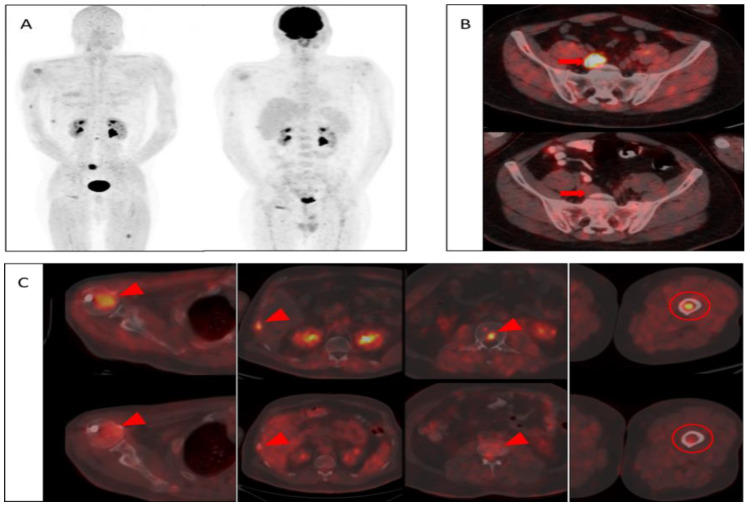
Patient No 3. 39-year-old male diagnosed with MM. (A) Visual comparison of maximum intensity projections of both modalities showed superior image quality and higher specific activity retentions in [68Ga]FAPI PET/CT (Left) without non-specific diffuse medullary uptake as in [18F]FDG PET/CT (Right). (B) In axial plane evaluations, both imaging methods demonstrated extramedullary involvement of MM in the presacral lymph node (Arrows); however, SUV_max_ value of the lymph node were noted to be significantly higher in [68Ga]FAPI PET/CT (Upper row). (C) In the visual evaluation of the bone lesions (Arrow heads), [68Ga]FAPI PET/CT (Upper row) demonstrated higher activity retentions in all locations with significantly lower background activity compared to [18F]FDG PET/CT (Lower row). Also, more pronounced focal activity retention was observed in the intramedullary region of the left femur with [68Ga]FAPI PET/CT (Upper row) compared to [18F]FDG PET/CT (Lower row), without morphological pathology (Circles).
